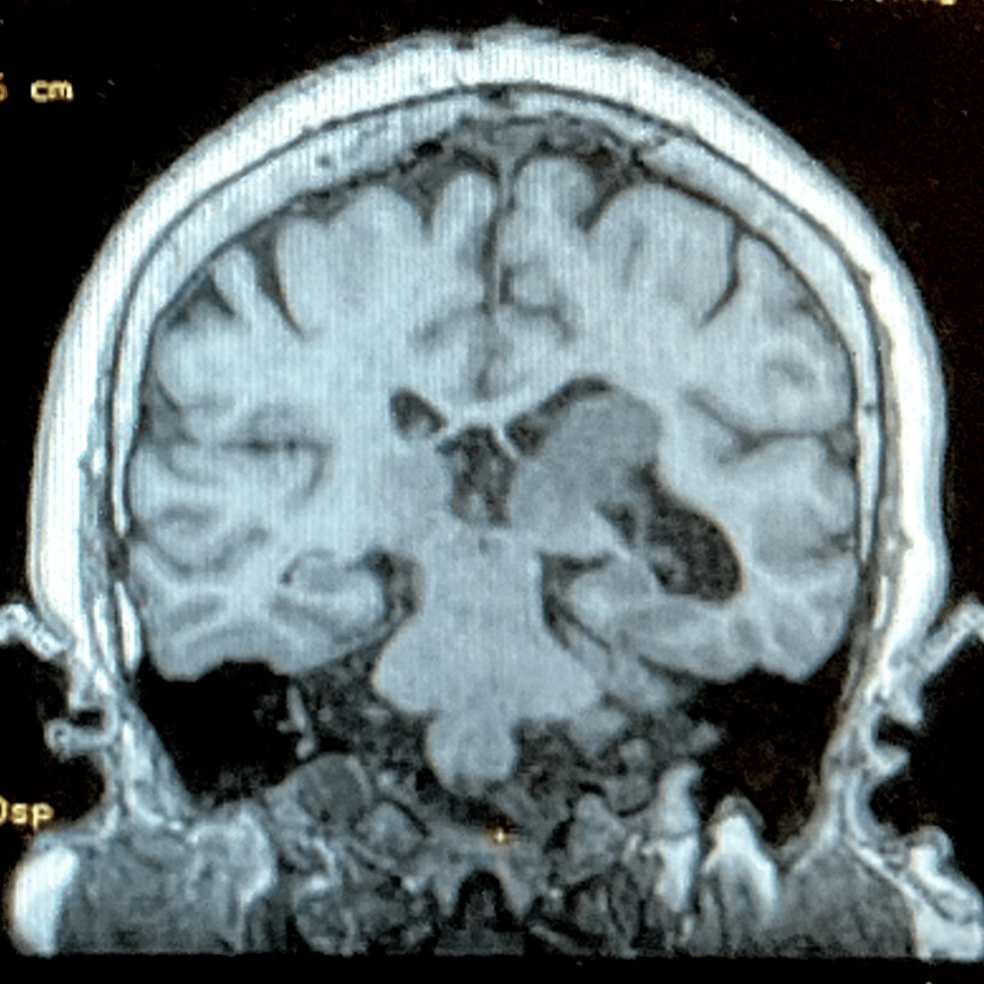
Magnetic resonance image demonstrating the lesion in the ventricle.
